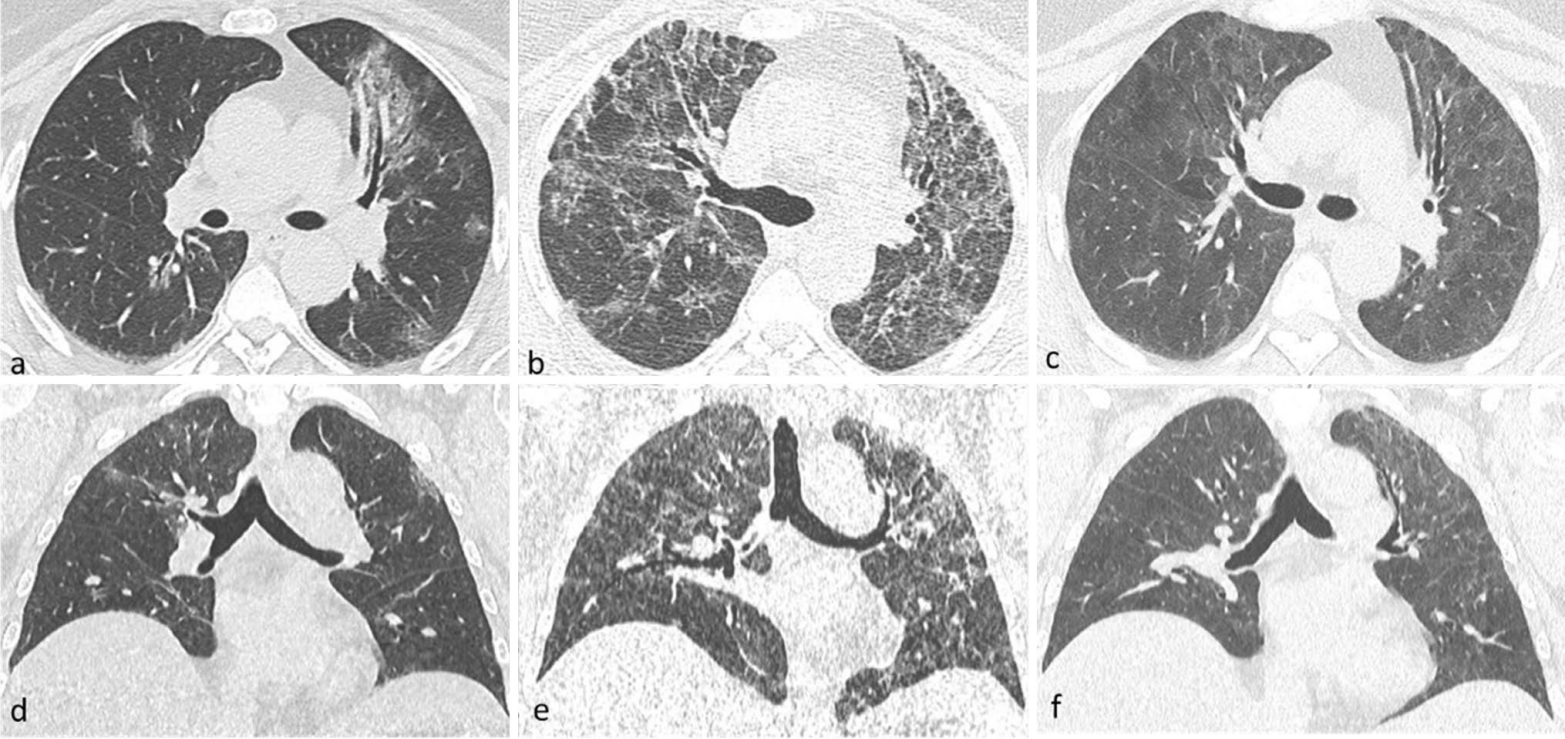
Disease evolution pattern consistent with OP sequelae. Axial (a–c) and coronal (d–f) CT images showing patchy ground glass opacities at baseline (a–d), diffuse ground-glass opacities with septal thickening, and perilobular opacities at 2–3 months (b–e), and subtle diffuse ground-glass with no CT features of lung fibrosis at 6–7 months (c–f).
